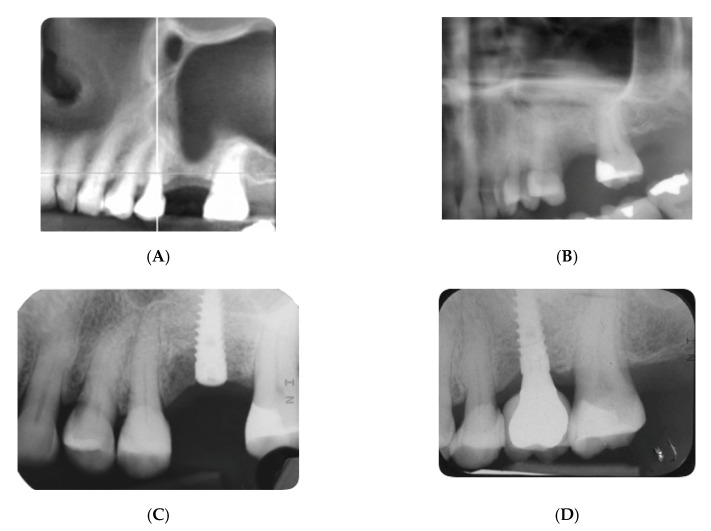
Radiographic images of a case with a residual bone height of 1 mm: (A) presurgical cone beam computed tomography (CBCT) showing the posterior residual bone; (B) image taken soon after the procedure of lateral sinus lift; (C) implant positioned after 5 months; (D) 1-year follow-up after loading.
